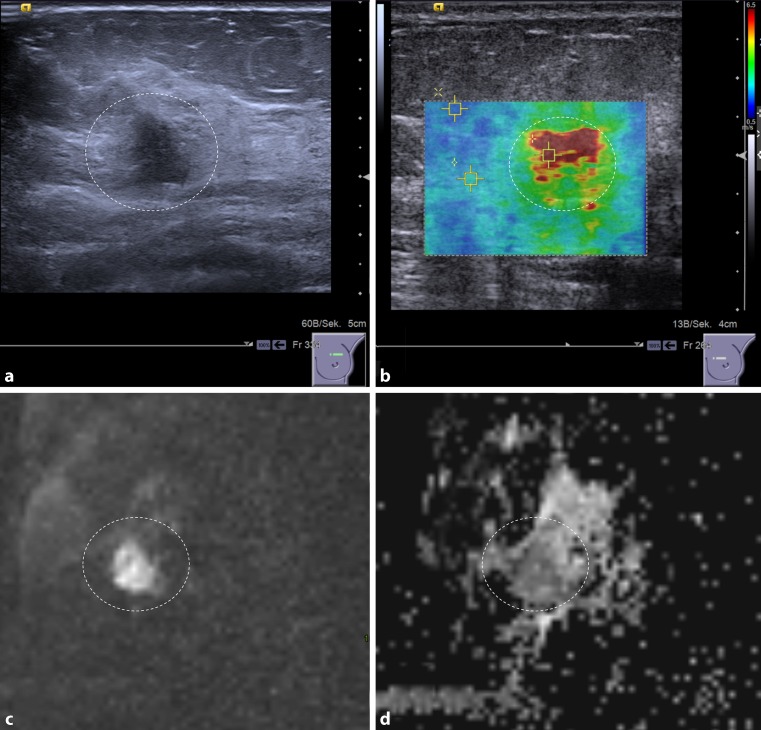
A 48-year-old woman with invasive ductal breast cancer, G3. The lesion (*dashed circle*) presents as an ill-defined hypoechogenic lesion on B‑mode ultrasound (a) that is associated with high SWV (4.6 m/s), coded *red* on the parametric ARFI overlay (b). The MRI-DWI scan of the same lesion shows a hyperintense lesion (c) corresponding to restricted diffusivity (1 * 10^−3^ mm^2^/s) that appears dark on the quantitative ADC map (d)
